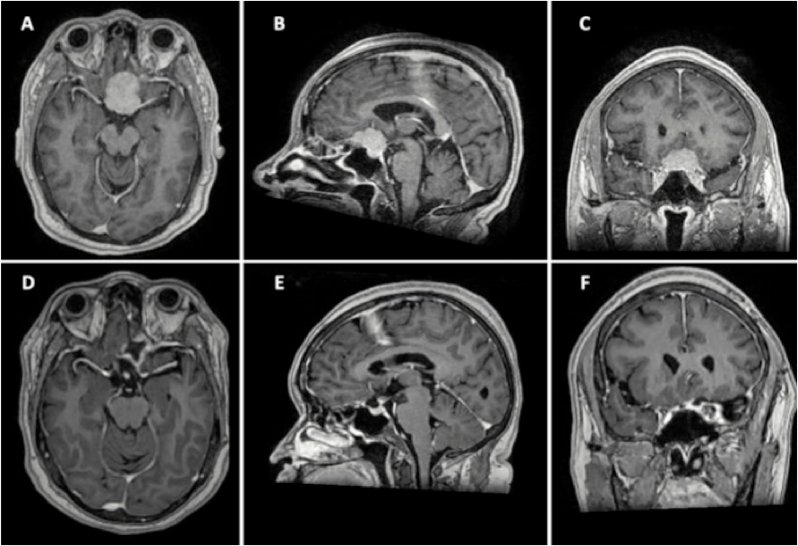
(A–C) Preoperative MRI: from left to right: axial, sagittal, and coronal views from a T1WI C+ showed an extra-axial lesion on the tuberculum sellae region, with suprasellar extension, and partial involvement of the left ICA. (D–F) Postoperative MRI: from left to right: axial, sagittal, and coronal views from a T1WI C+ in which gross total resection is observed.
